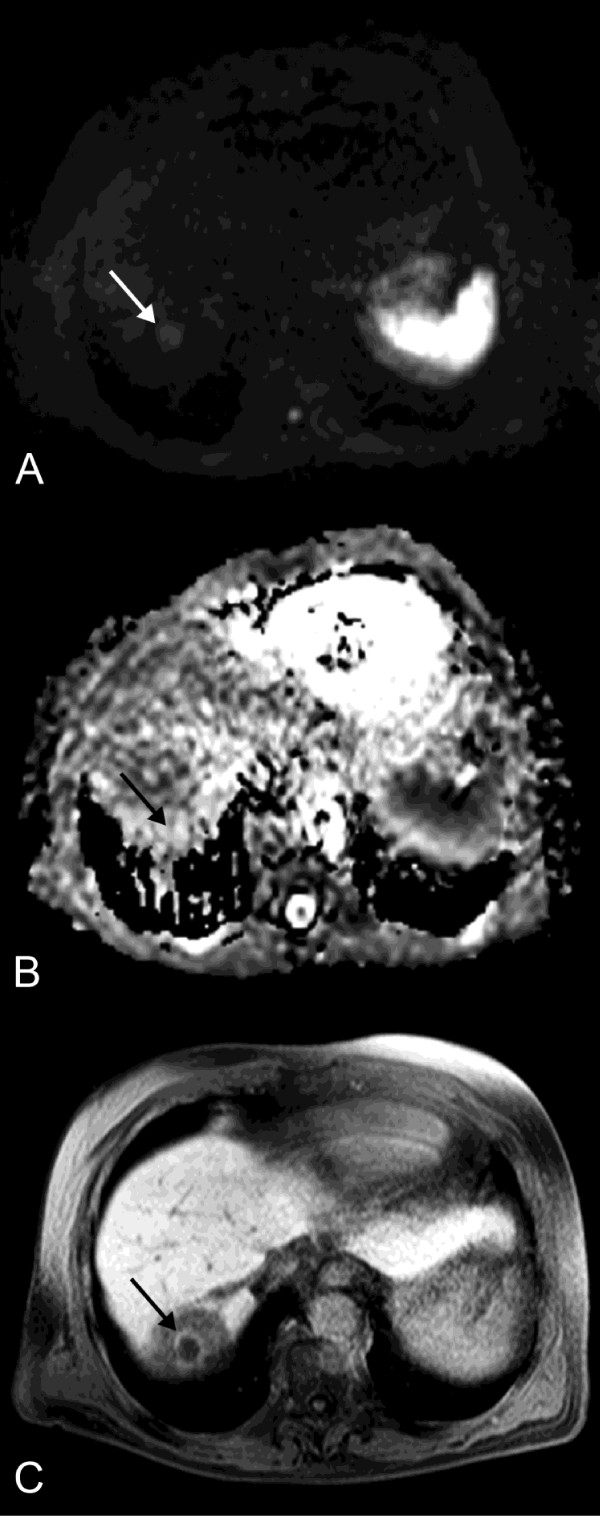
Follow-up MRI. DWI (A) and ADC map (B) performed 105 days post intervention (same patient as in Figure 1) show a rise of mean tumor ADC of 75.2% to 2.26 × 10^-3 ^mm^2^s^-1 ^(arrow). This finding correlates with a decrease in tumor volume by 90.6% (2.2 cm^3^), depicted in T1w Gd-EOB-DTPA enhanced MR image in hepatobiliary phase (C). The circular hypointense region around the treated lesion in (C) indicates the area of irradiation induced reversible hepatocyte dysfunction.
